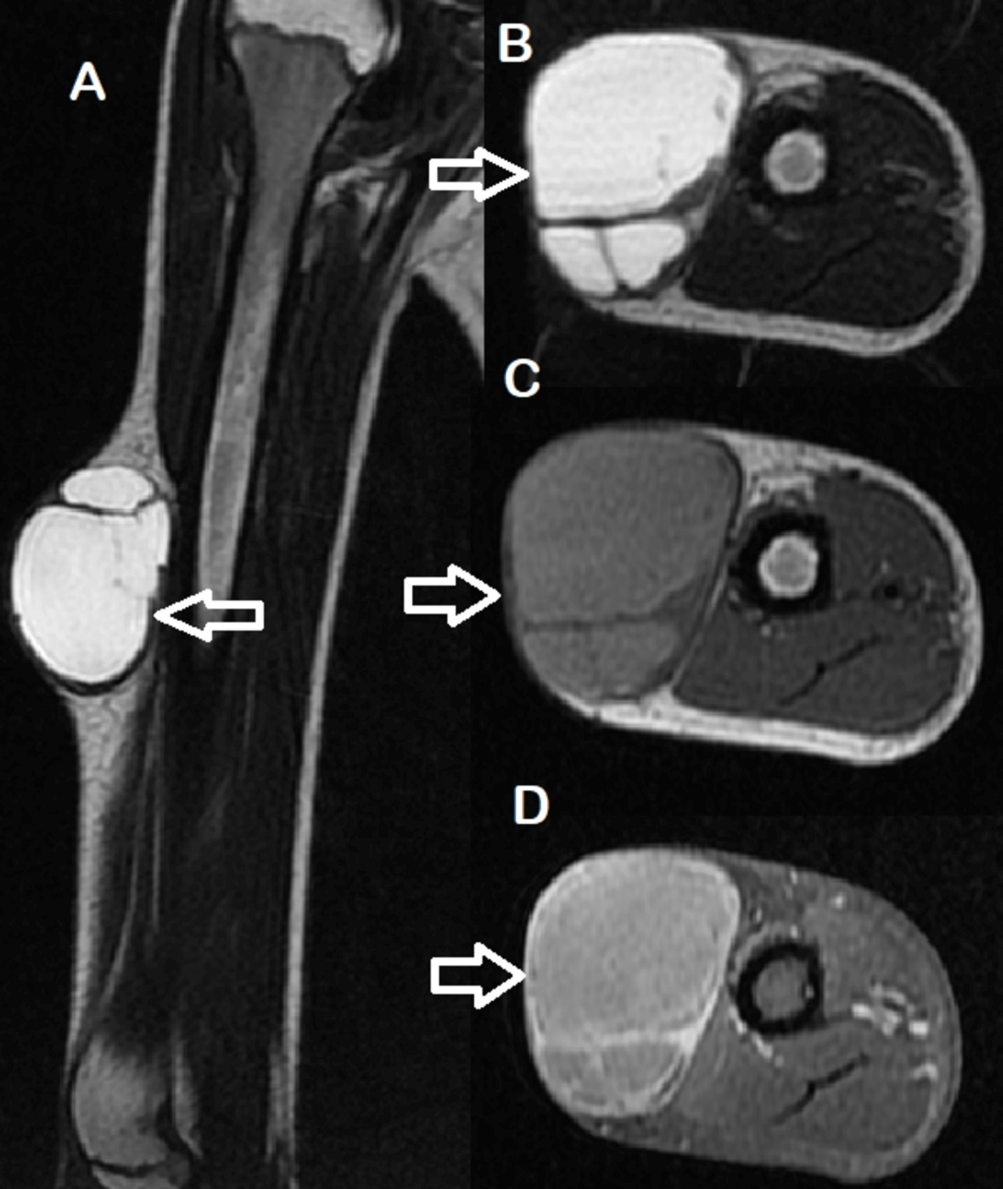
A, B: Coronal and transverse magnetic resonance imaging slices the lesion exhibiting cystic transformation, demonstrating a marked hyperintensity on T2 (white arrow) with internal septum and low-signal-intensity capsule. C: Transverse magnetic resonance imaging showing right arm superficial mass exhinbiting mild hyperintensity signal on T1 (white arrow) with a low-signal-intensity capsule. D: Transverse magnetic resonance imaging showing enhacement limited to the peripheral wall and septum and following contrast administration (white arrow).
